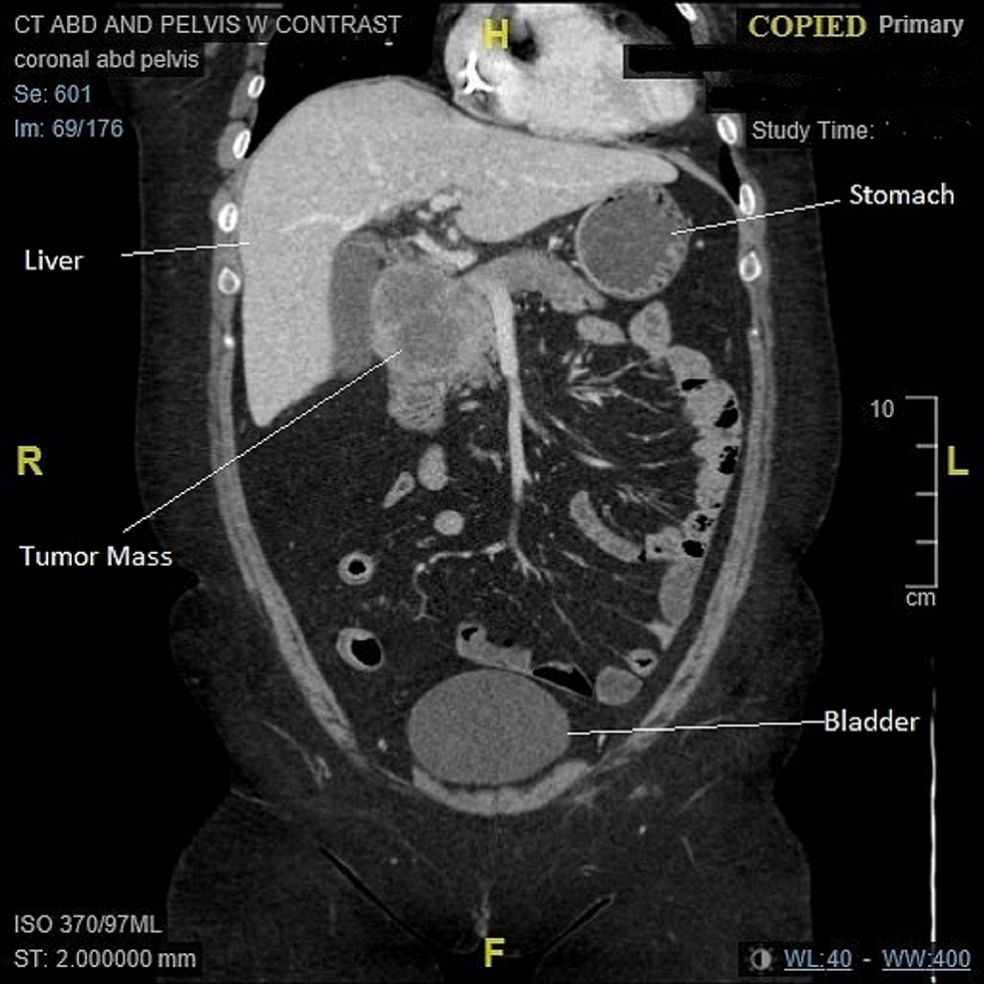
CT abdomen (coronal plan): tumor mass in topography of head of the pancreas.
